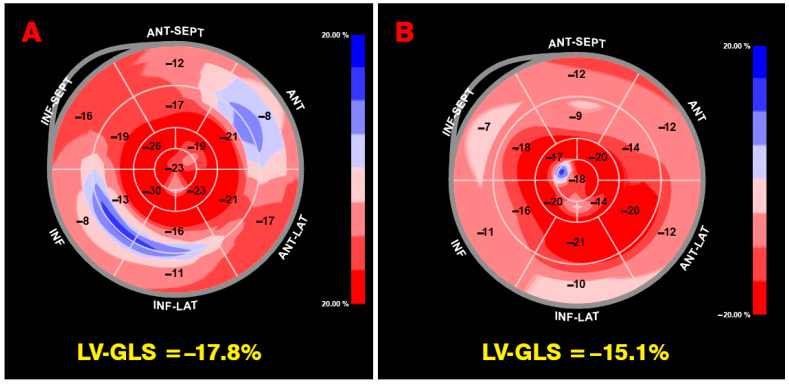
Representative 2D speckle-tracking echocardiography–derived left ventricular global longitudinal strain bull’s-eye maps in a healthy individual with mitral valve prolapse (A) and a patient with pectus excavatum (B). In both cases, the LV-GLS bull’s-eye maps demonstrate reduced basal segment deformation with relative preservation of apical strain, resulting in an apparent apical sparing pattern. These findings highlight the potential influence of thoracic morphology on regional myocardial deformation patterns independent of myocardial infiltration.
